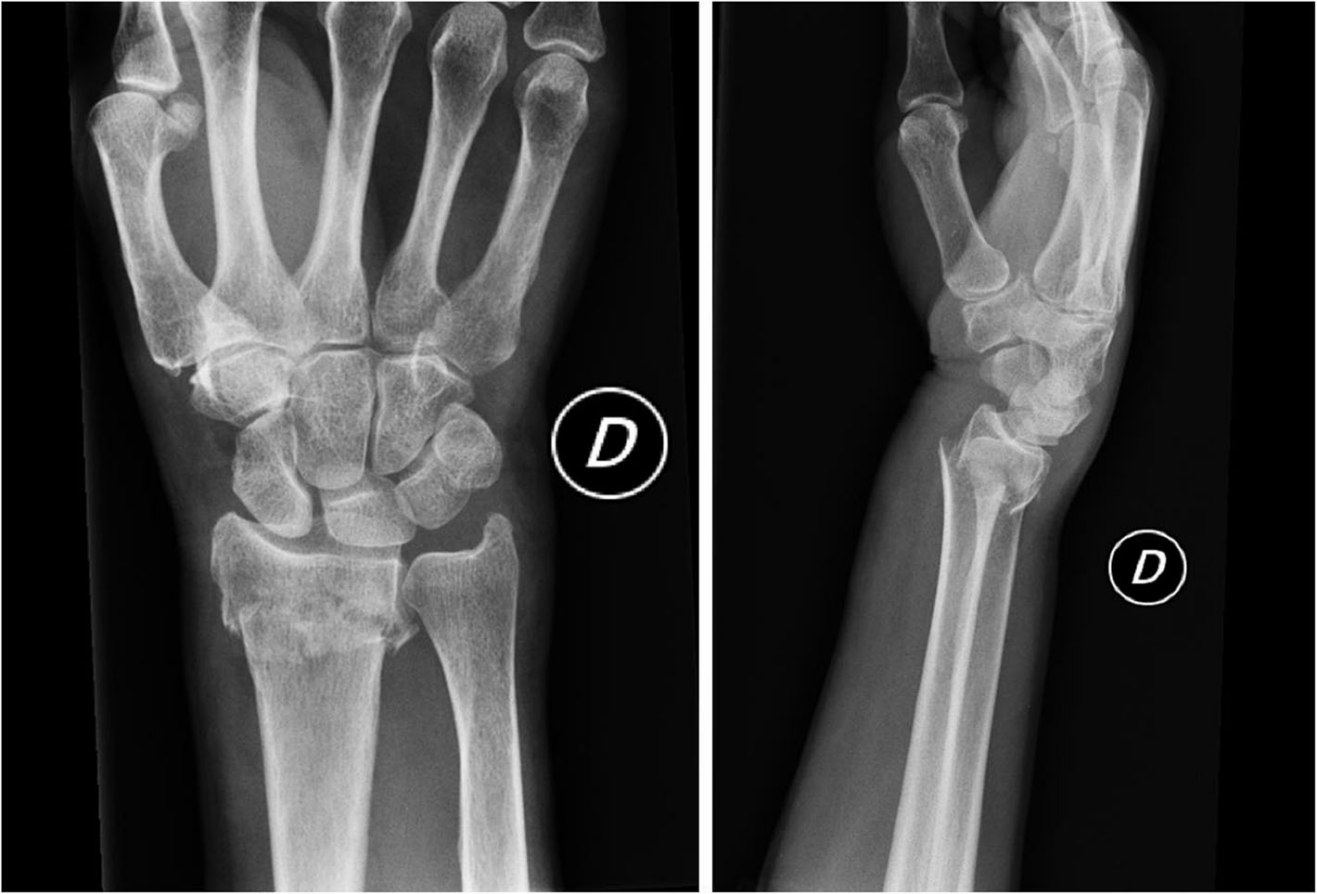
Postero-anterior and lateral view radiographs showing extra-articular fractures of the distal radial epiphysis with dorsal displacement (type 2R3A2.2 according to the AO/OTA classification)
